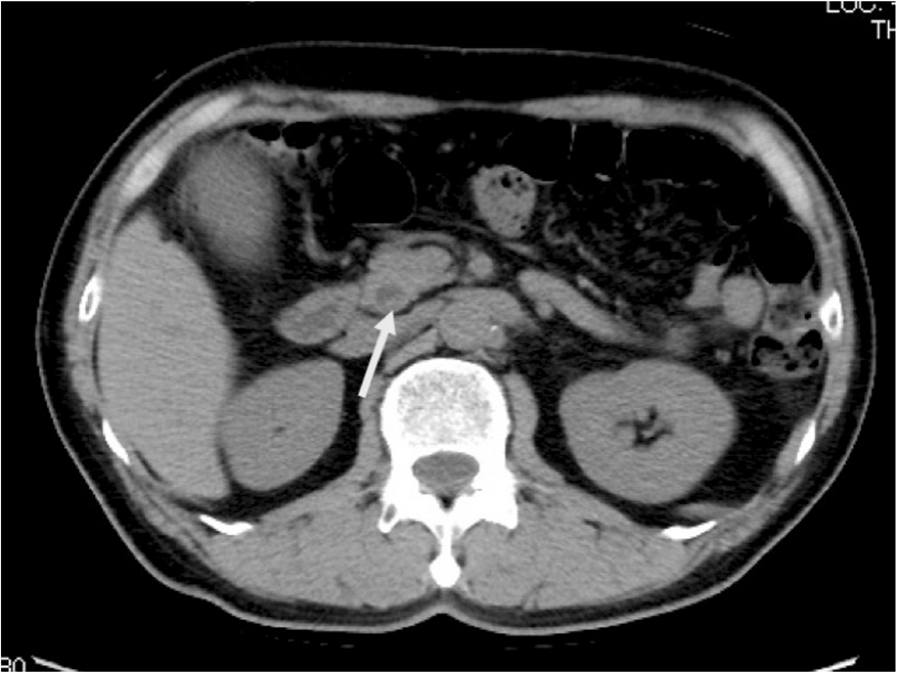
Abdominal contrast-enhanced CT showed the slightly dilated CBD and a high-density spot (arrow) in the DBD, suggesting CBD stone
